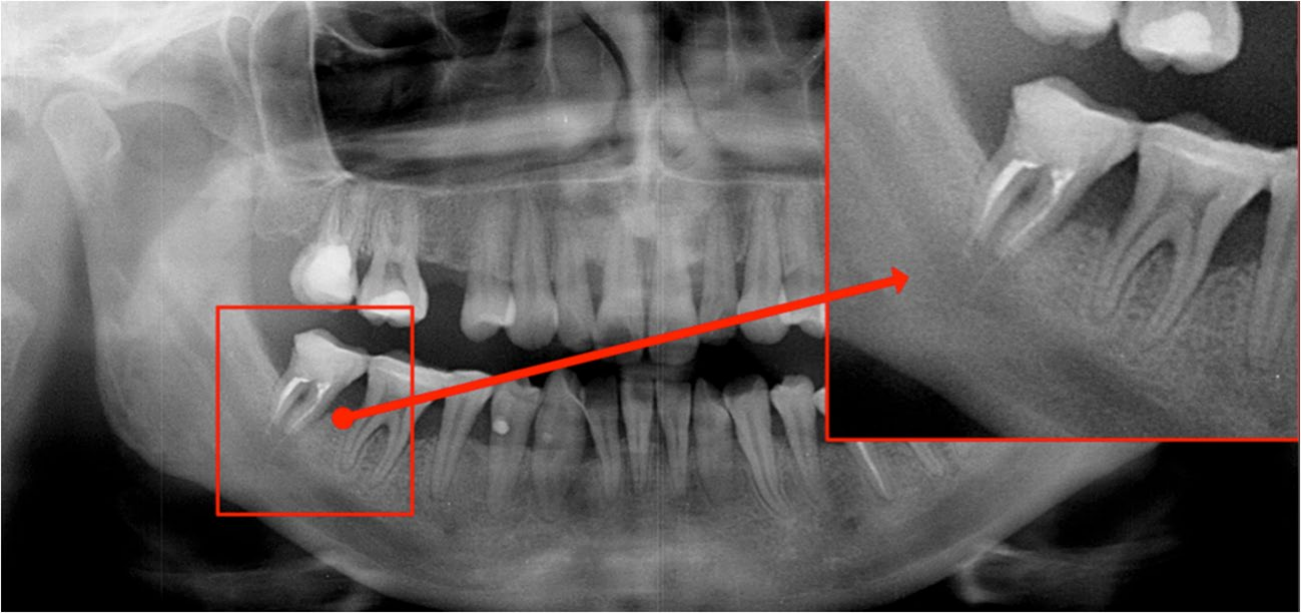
Panoramic radiograph of a patient where root filling material was accidentally overfilled into the nerve canal in the mesial root of tooth 47. This resulted in recurrent neuropathic pain, which disappeared after the removal of the material
